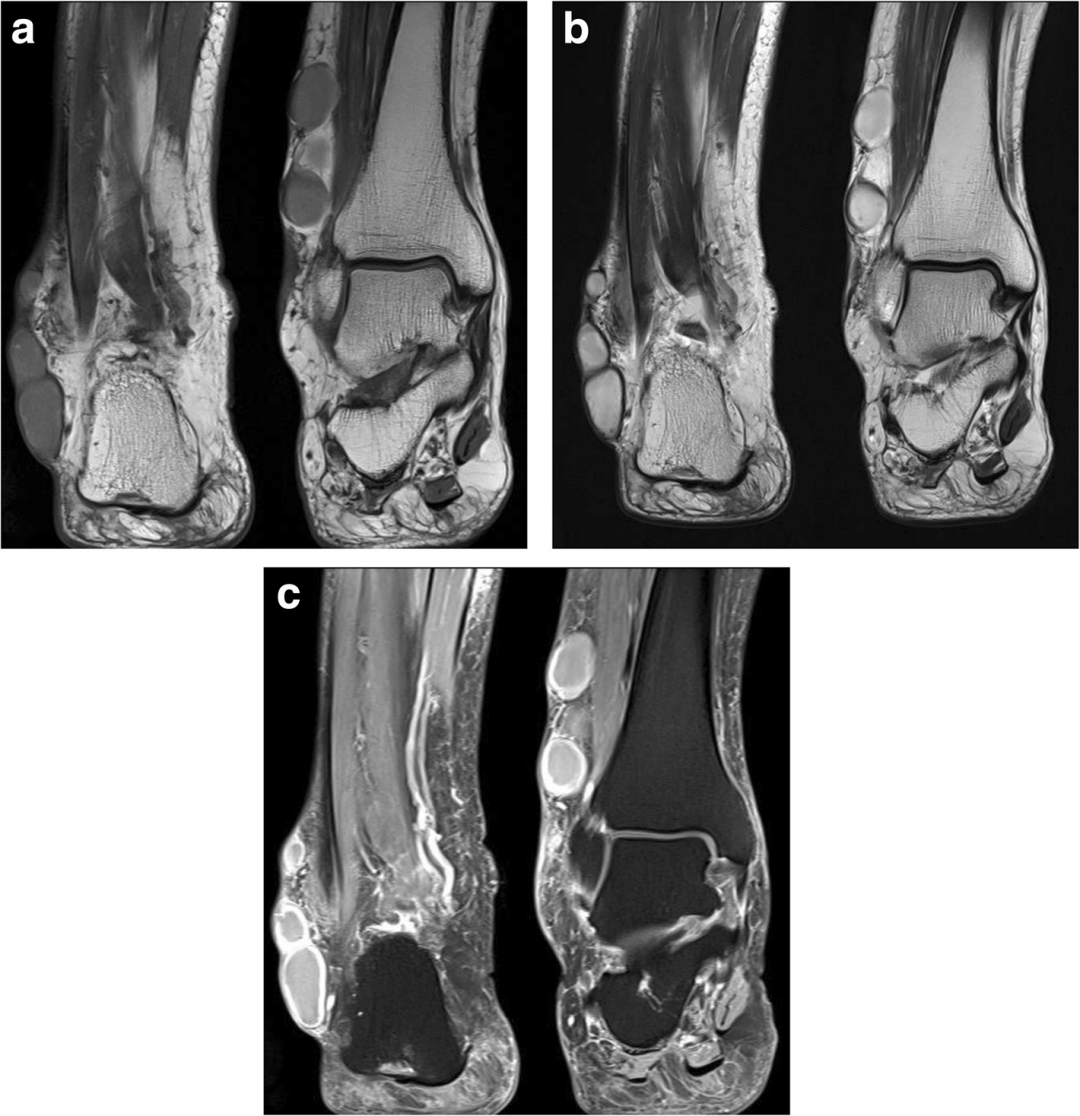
Magnetic resonance imaging of the right ankle at admission: (a) T1-weighted, (b) T2-weighted, and (c) fat-suppressed T1-weighted images
